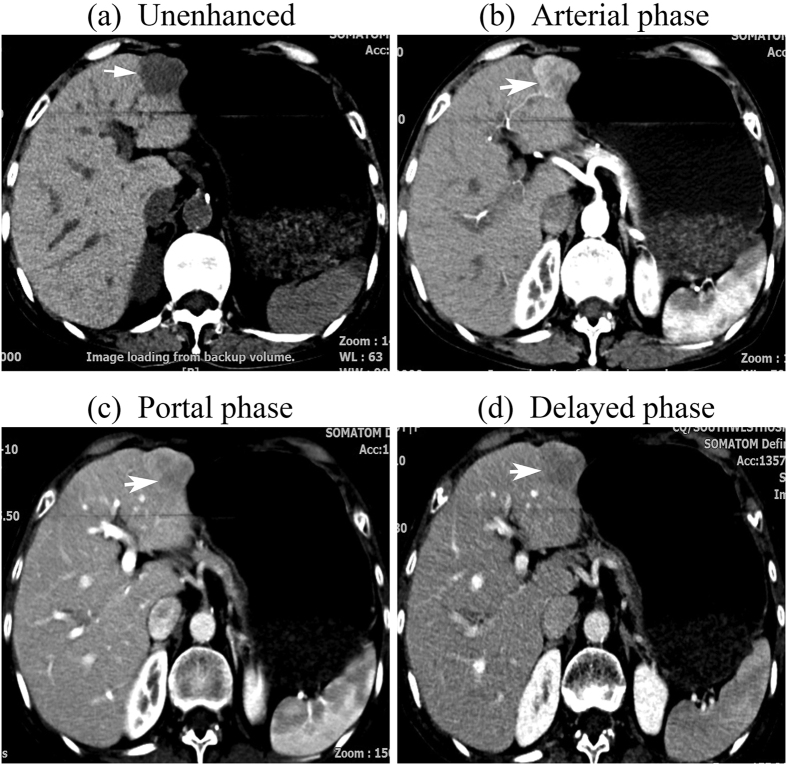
tif CT images of a 69-year-old woman with a 2.7-cm –diameter ICC
